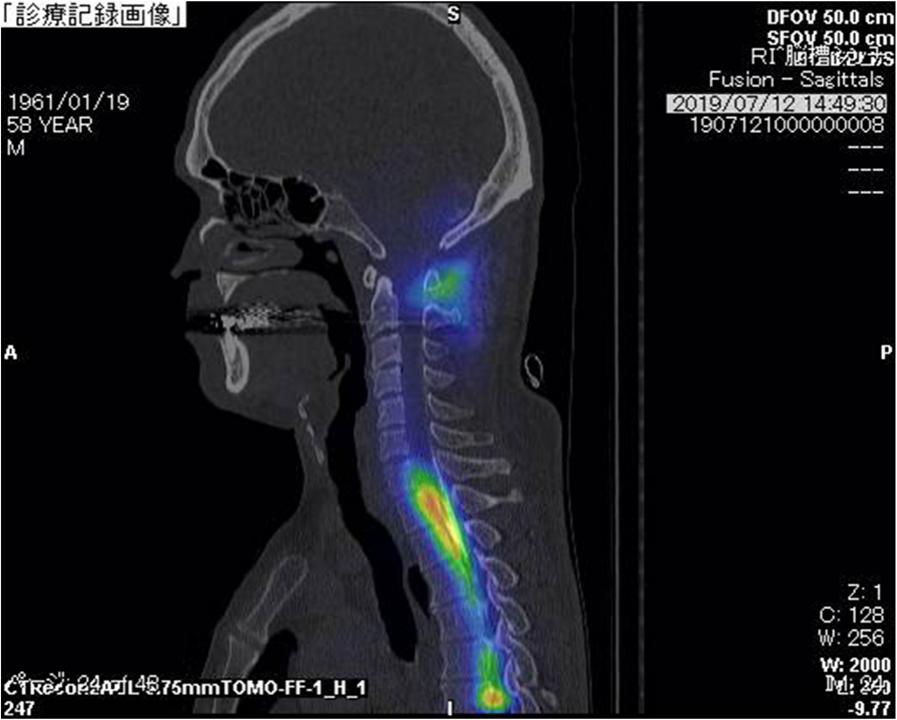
CSF leakage detected by cisternoscintigram. A saggital view of cisternoscintigram in the cervical level demonstrated CSF leak in the retrospinal region at C1-C2 level
